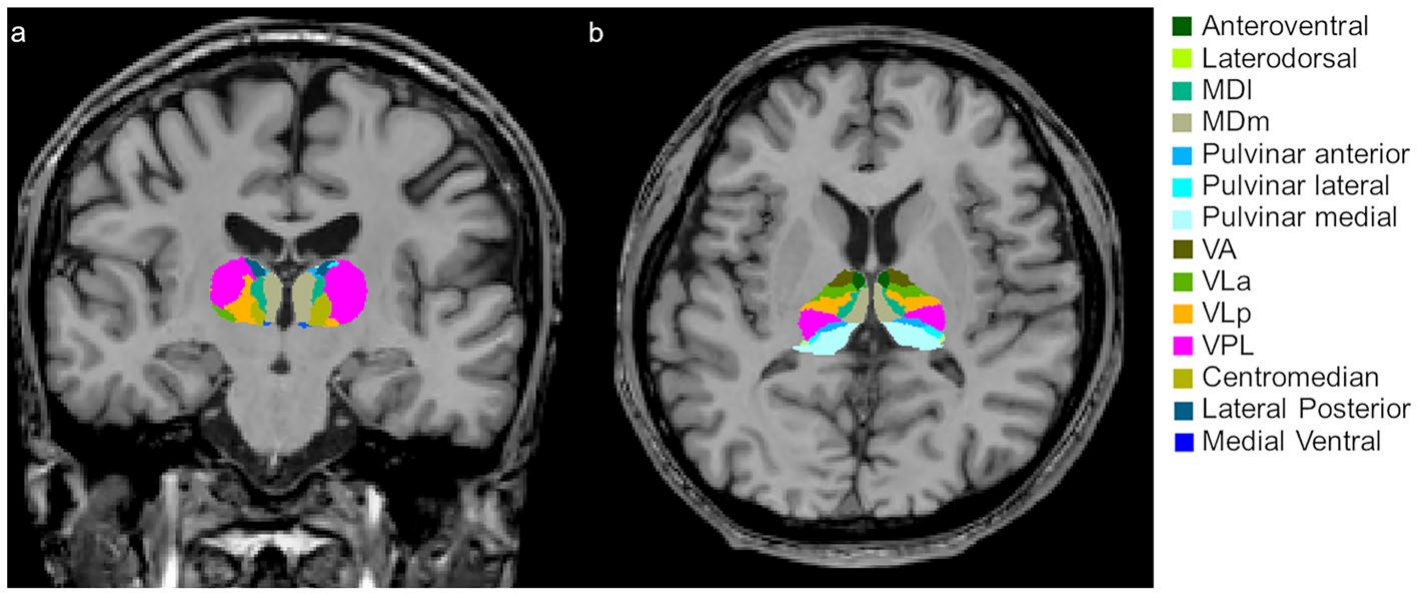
Example of thalamic nuclei segmentation. Segmentations and labels of thalamic nuclei in the coronal (a) and axial (b) plane generated by FreeSurfer (not all segmentations are shown). The segmentations are overlaid on the T1-weighted scan. This figure is generated from our previous study^17^. *MDl* Mediodorsal lateral parvocellular nucleus, *MDm* Mediodorsal medial magnocellular nucleus, *VLa* Ventral lateral anterior nucleus, *VLp* Ventral lateral posterior nucleus, *VPL* Ventral posterolateral nucleus, *VA* Ventral anterior nucleus.
